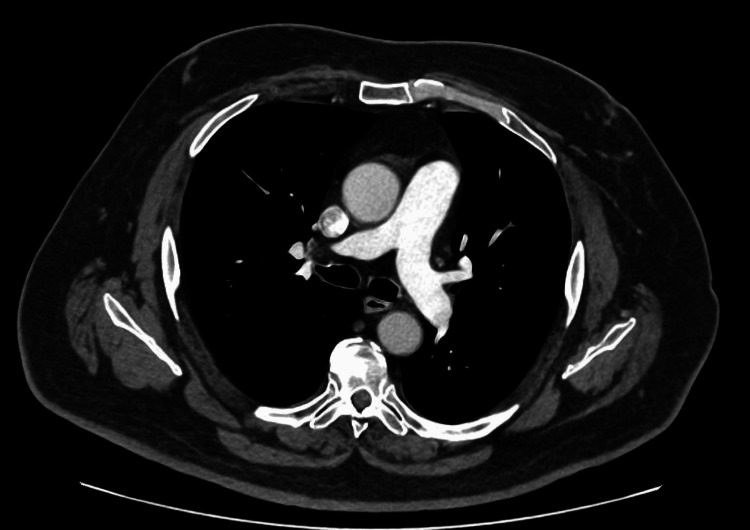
CT Thorax – Mediastinum/Aortic Arch Level CT thorax at the level of the aortic arch demonstrating clear anterior mediastinum with no evidence of thymoma, an important consideration in the evaluation of myasthenia gravis.
